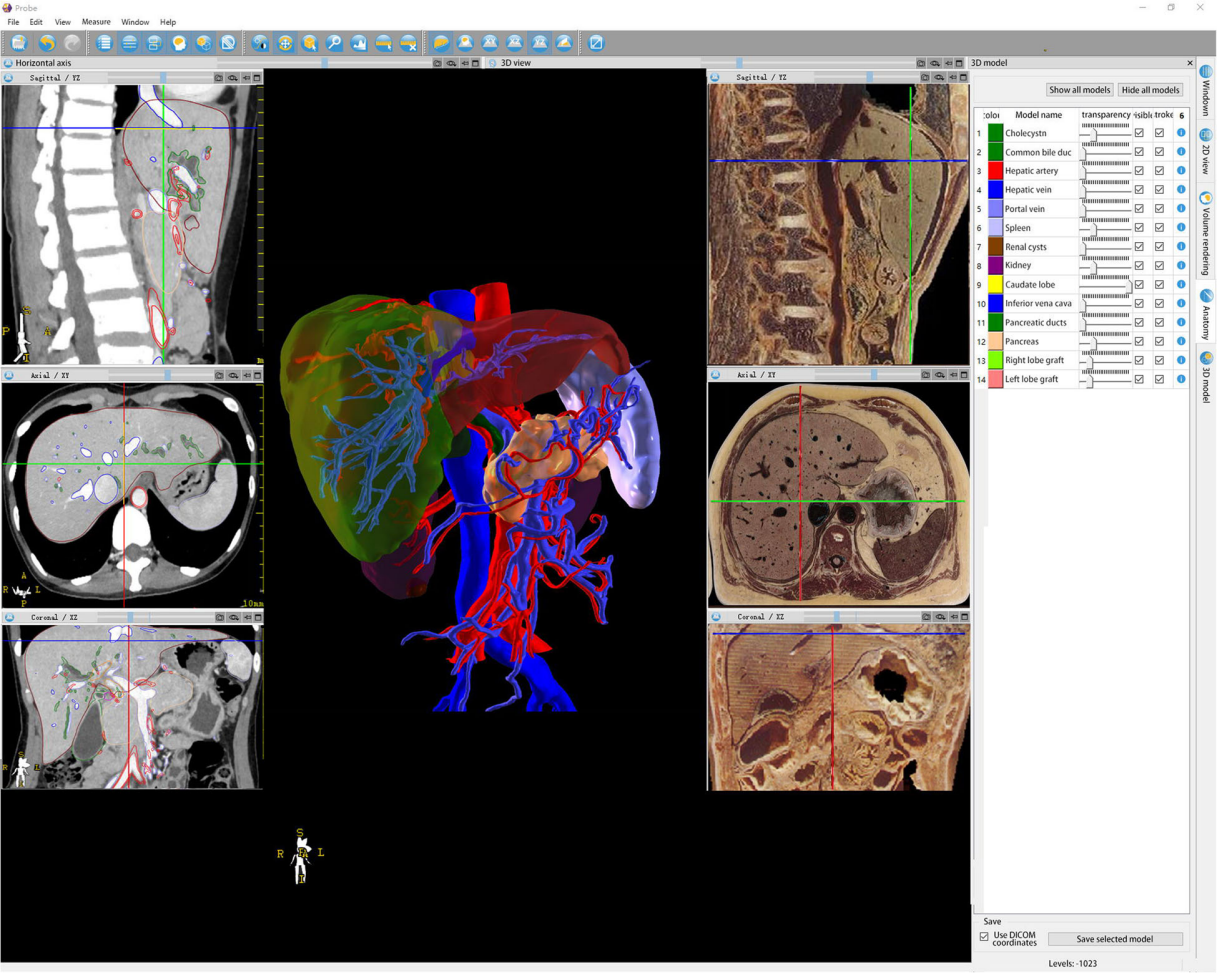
Liver CT and corresponding sectional anatomy. The left side is the liver CT, the right side is the corresponding sectional anatomy, and the middle panel is the 3D liver and blood vessels reconstructed by CT. It can be rotated and magnified arbitrarily
